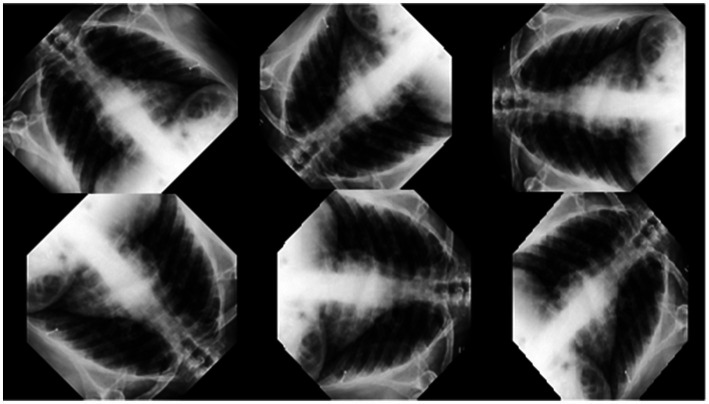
Reproduction of X-ray data.
